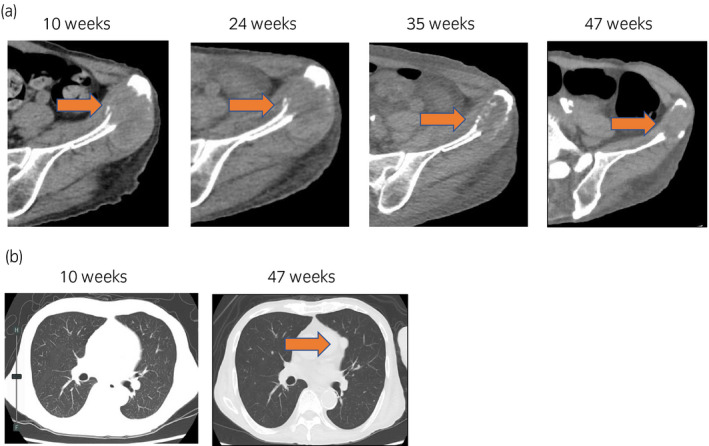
Sequential CT scans after discontinuation of combination therapy. (a) Abdominal CT scans show that the metastatic site of iliac bone maintained partial response for 30 weeks after the discontinuation of combination therapy without any additional therapy. After 41 weeks after discontinuation follow‐up, CT showed reduction of iliac bone metastatic sites. (b) Chest CT scan shows the new lung metastatic lesion at 41 weeks after discontinuation and the patient was diagnosed as progressive disease. The number of weeks indicates duration after the first initiation of combination therapy.
